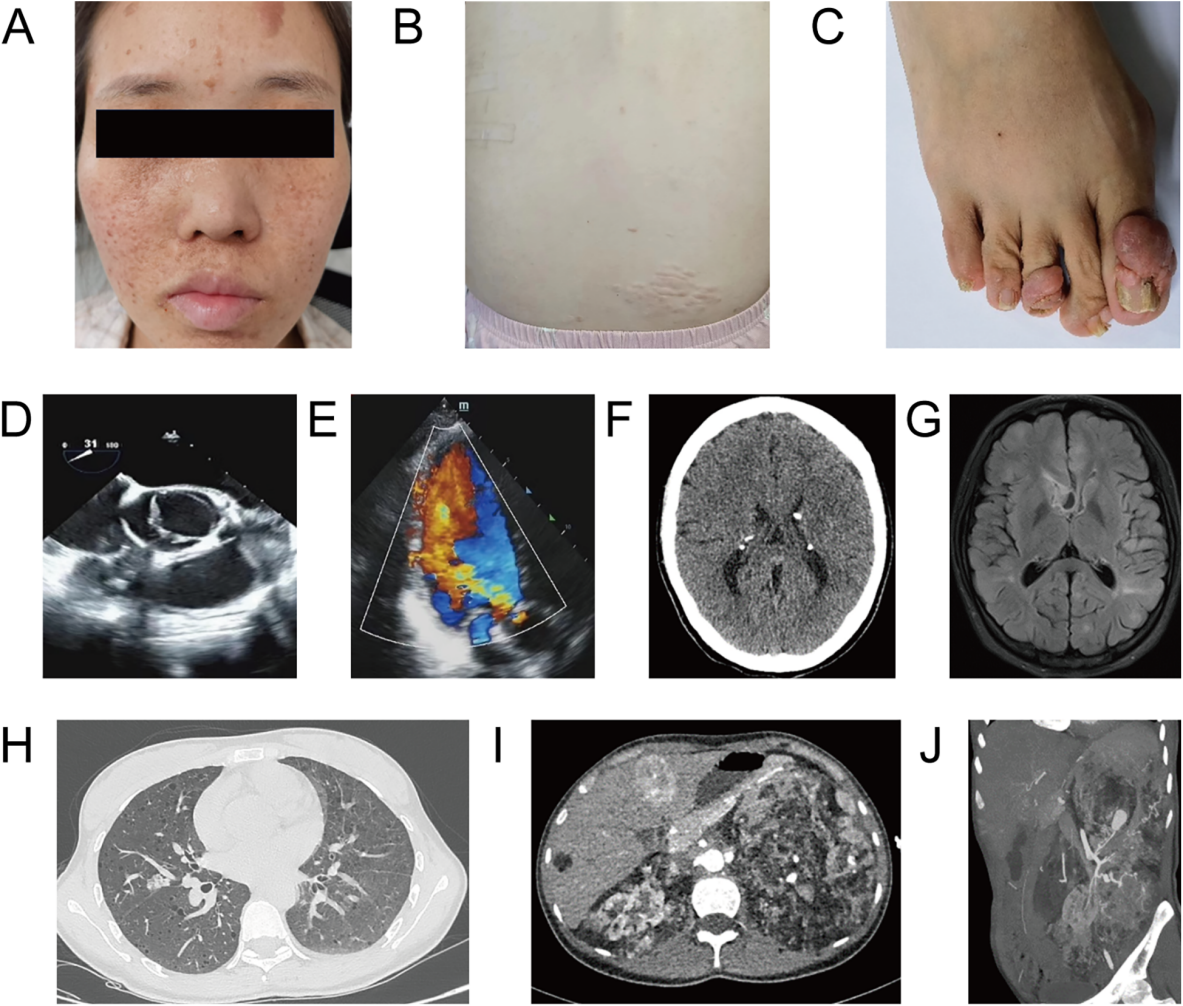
Major clinical and imaging findings in a 26-year-old woman with TSC. (A) Facial photograph demonstrates multiple angiofibromas on her face. (B) A shagreen patch on the right waist. (C) Subungual fibromas affecting toenails. (D,E) Echocardiogram showing a BAV with lobe thickening and severe aortic valve insufficiency. (F) Head CT demonstrating bilateral calcifications in the lateral ventricles and a nodular calcification. (G) Brain T2-weighted FLAIR sequence MR showing multiple cortical and subependymal nodules. (H) Chest CT revealing multiple cystic lesions and a well-circumscribed nodule in the right middle lobe. (I) Epigastrium postcontrast CT suggesting rapid arterial phase enhancement and delayed washout in liver S4 and fatty nodule without enhancement in S6. Both kidneys are enlarged (left more prominent) with multiple fat-containing lesions. (J) MIP image from a CT angiogram of the left kidney demonstrating numerous tortuous, narrowed, and dilated arterioles.
